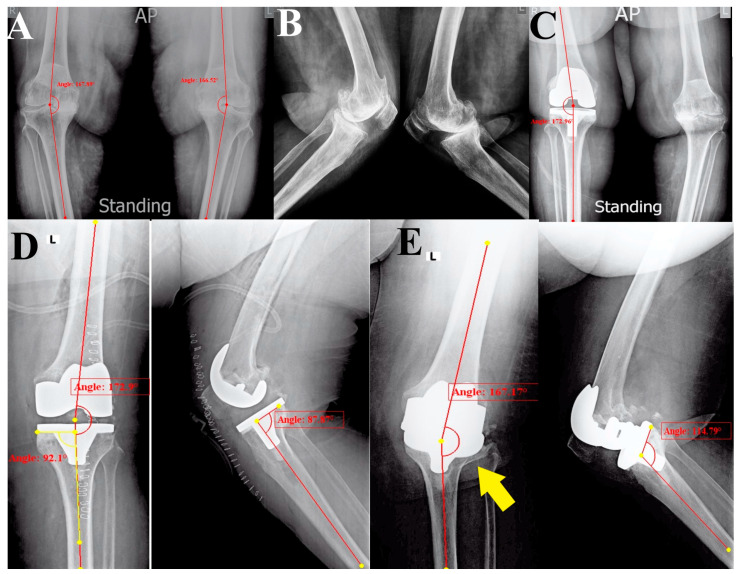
A series of initial radiographic evaluations (anteroposterior (AP) and lateral views); (A,B) the initial presentation had been more than four years ago with bilateral tricompartmental knee advanced osteoarthritis associated with varus deformity. (C) After having right knee TKA using a posterior stabilized prosthesis (four years ago). (D) Immediate postoperative radiographs after left TKA showed acceptable limb and individual implant alignment and positioning. (E) Three weeks postoperatively, catastrophic failure of the tibial component with lateral tibial plateau fracture (yellow arrow) and loosening of the tibial component.
